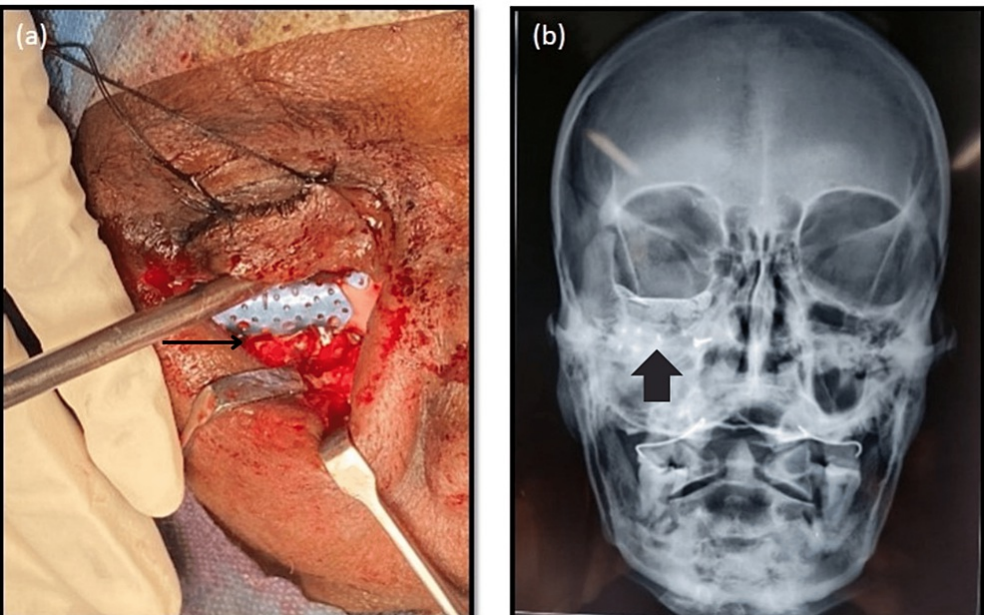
Intraoperative and postoperative pictures of the patient: a) intraoperative: contouring and securing the orbital floor mesh; b) postoperative: X-ray showing titanium mesh placed over the right orbital floor.
